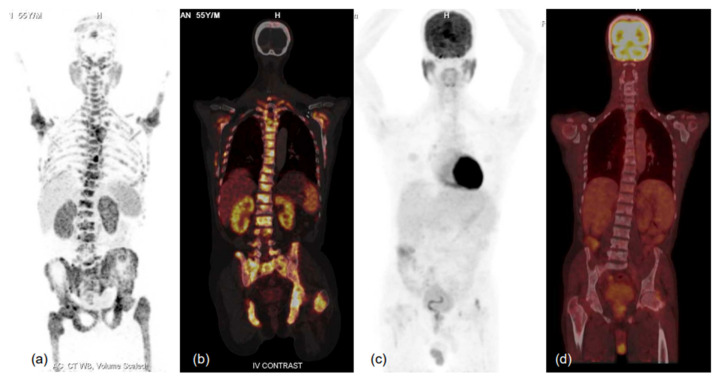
MIP images of ^68^Ga-PSMA-11 (a,b) and FDG (c,d) PET-CT scans and corresponding fused coronal images (b-^68^Ga-PSMA-11 and d-FDG PET-CT scans) in bone window showing PSMA-expressing multiple sclerotic and marrow lesions involving almost the entire axial and proximal appendicular skeleton, while no significant FDG uptake (metabolic activity) is evident in the lesions. Such patients respond well to therapy, especially PSMA-targeting radionuclide therapy, and demonstrate relatively favourable prognoses in terms of survival and quality of life benefits. Thus, dual-tracer PET using FDG and ^68^Ga-PSMA-11 helps in patient selection for PSMA peptide receptor radionuclide therapy (PRLT) and in predicting treatment outcomes. He was scheduled for ^177^Lu-PSMA-617 therapy, but unfortunately could not attend due to logistical constraints.
